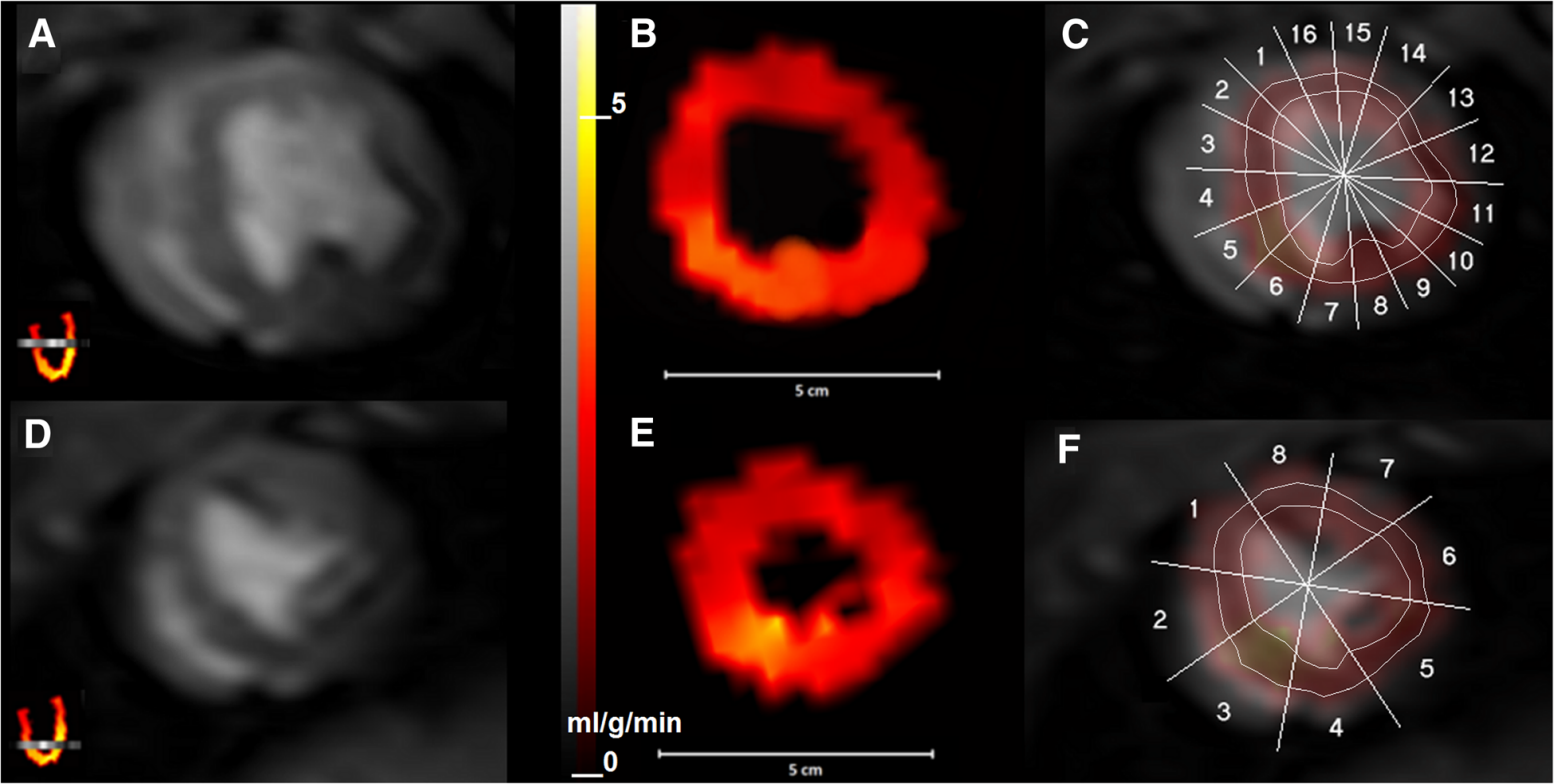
MRI-, PET- and co-registered images of the short axis slice. The mid-ventricular short axis slice and (upper row) and the apical short axis slice (lower row). a and d MR images, b and e PET images, c and f co-registered short axis MRI- and PET-images with ROIs. Orientation of the MRI slices is indicated in long axis PET images in the lower left corners of the MR images. MR images were acquired using the T1-TFE sequence. Contrast agent can be seen in the right and left ventricles
